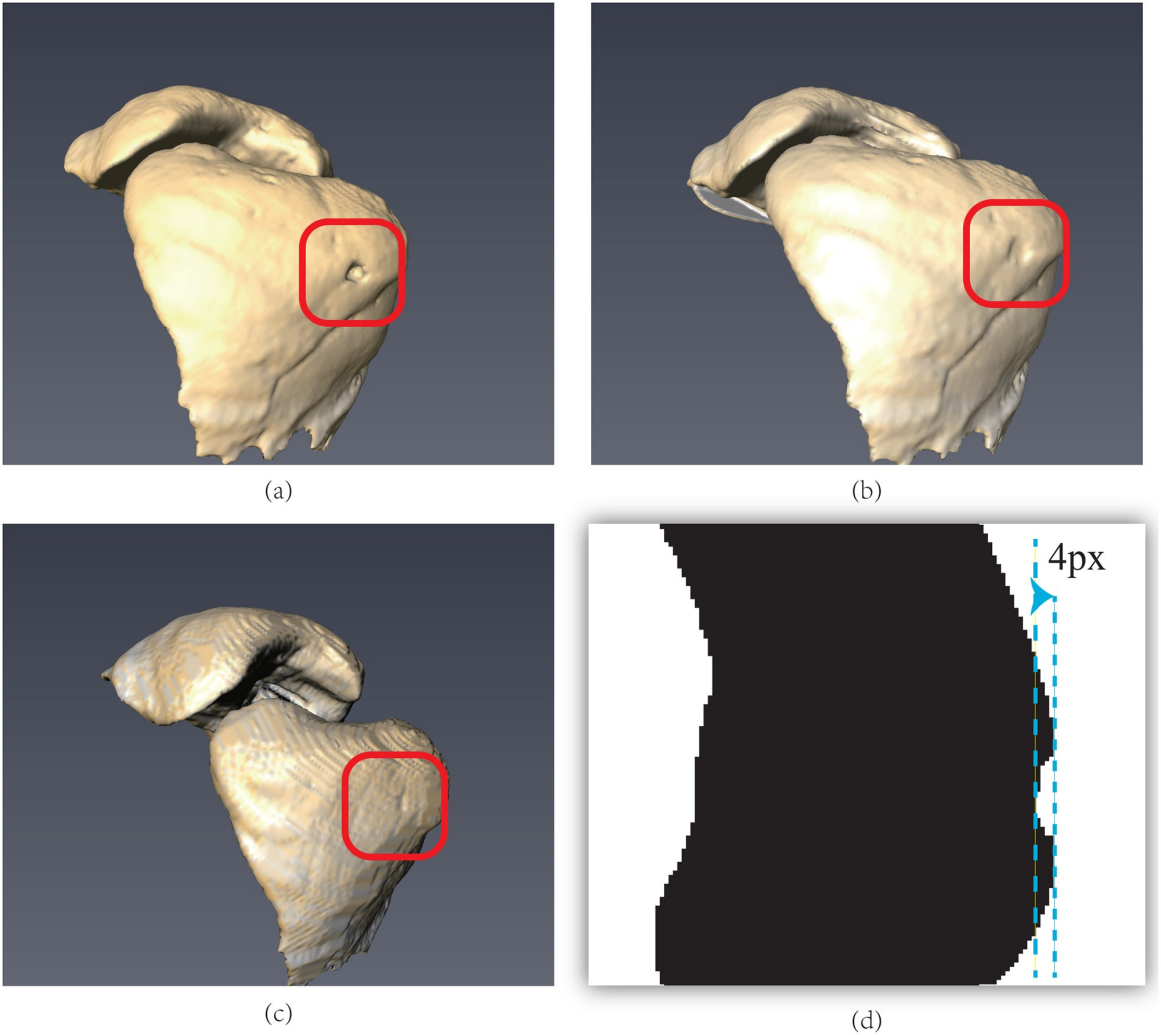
The comparison over 3D reconstruction models. (a) The model based on original CT slices; (b) the 3D model of this manuscript; (c) the reconstruction model of [18]; (d) the error of the algorithm in this paper.
